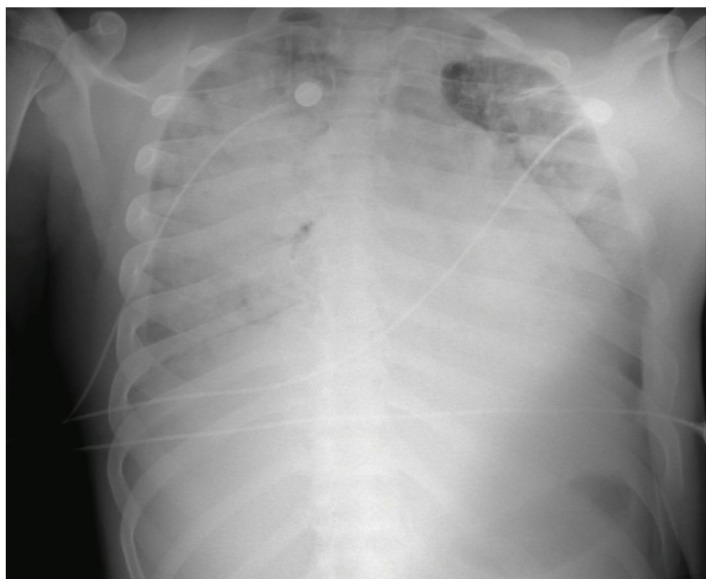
Post-intubation chest X-ray. Anteroposterior chest radiograph showing diffuse bilateral alveolar opacities with loss of lung aeration, consistent with severe acute respiratory distress syndrome (ARDS). Presence of endotracheal tube confirmed in proper position.
